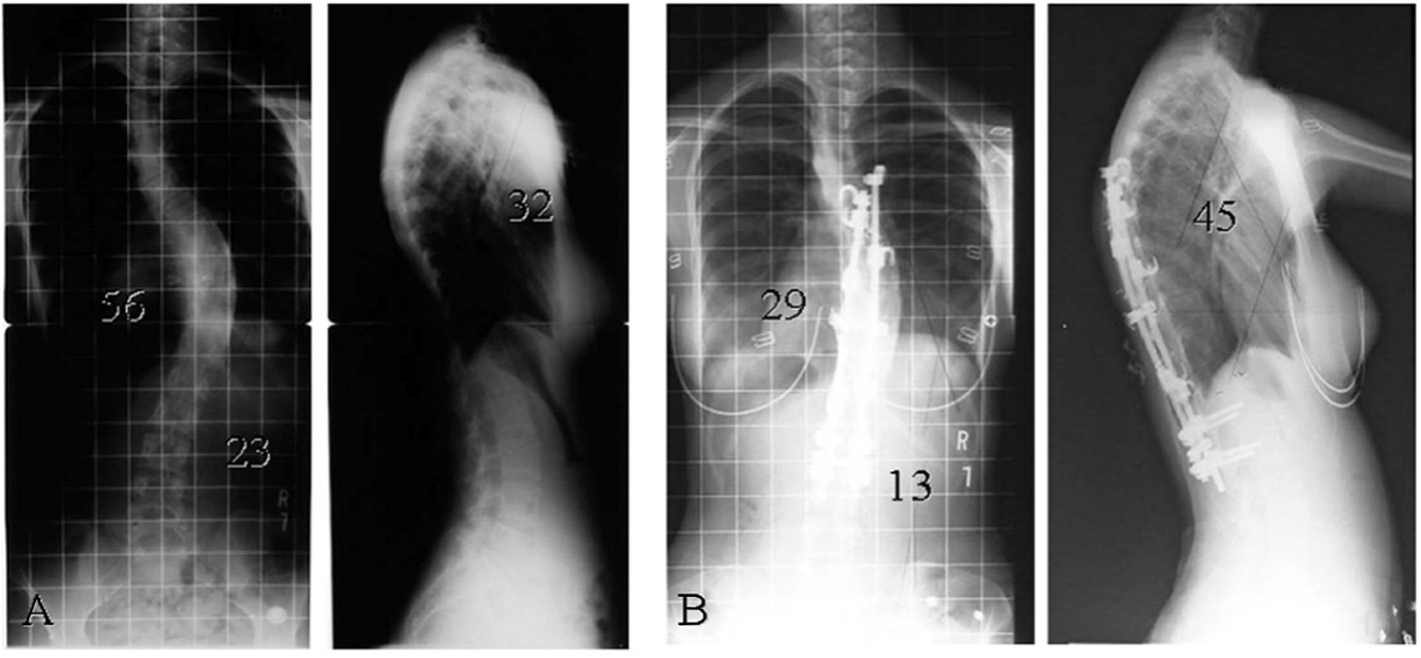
Standing radiographs. (A) Radiographs before initial surgery. (B) Radiographs 3 years after initial surgery.
